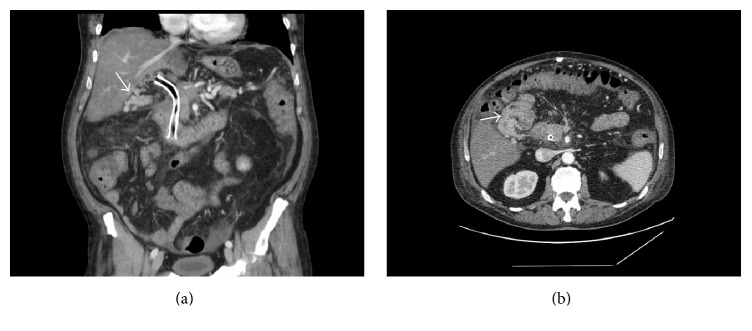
Abdominal contrast-enhanced CT. (a) Oblique plane, showing gallbladder varices, and (b) axial plane, showing gallbladder varices and cholecystoenteric anastomosis.
